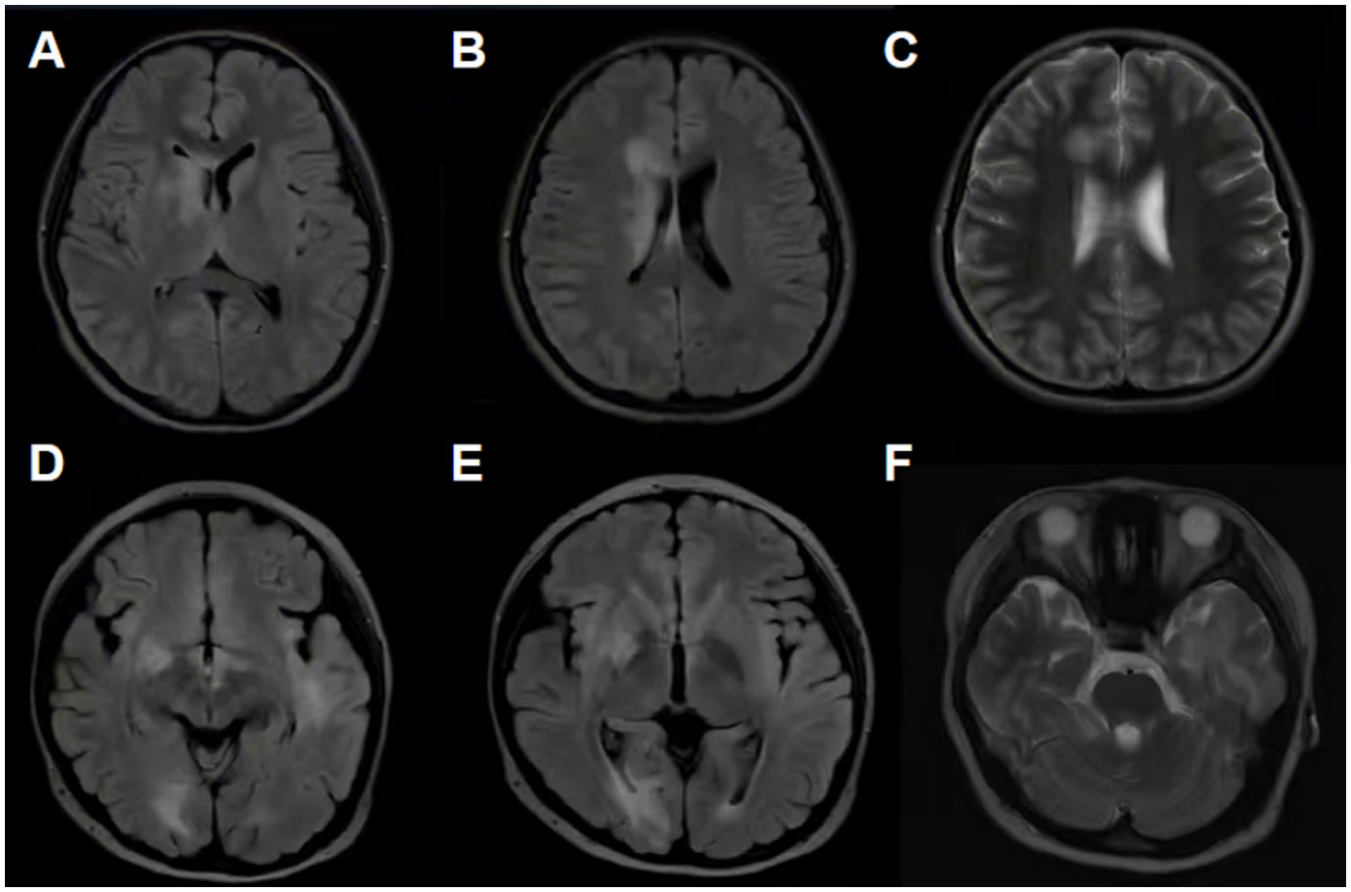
(A–C) 11 year-old girl; (D–F) 12 year-old girl. (A) FLAIR sequence shows patchy hyper-intense signals in the right basal ganglia and the genu of the corpus callosum. (B) FLAIR sequence shows patchy hyper-intense signals adjacent to the right lateral ventricle and in the body of the corpus callosum. (C) T2 sequence shows patchy hyper-intense signals in the deep cerebral white matter of the right frontal lobe. (D) FLAIR sequence shows patchy hyper-intense signals in both temporal lobes and the right occipital lobe. (E) FLAIR sequence shows patchy hyper-intense signals in the right basal ganglia and the right occipital lobe. (F) T2 sequence shows extensive patchy hyper-intense signals in the left temporal lobe.
